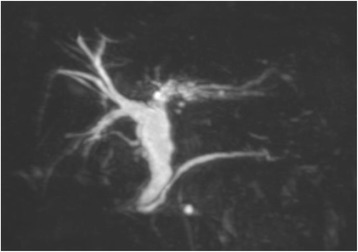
MRI showed dilated extra hepatic bile duct and intrahepatic bile duct in left liver and no finding of anomalous connection with pancreatic duct
